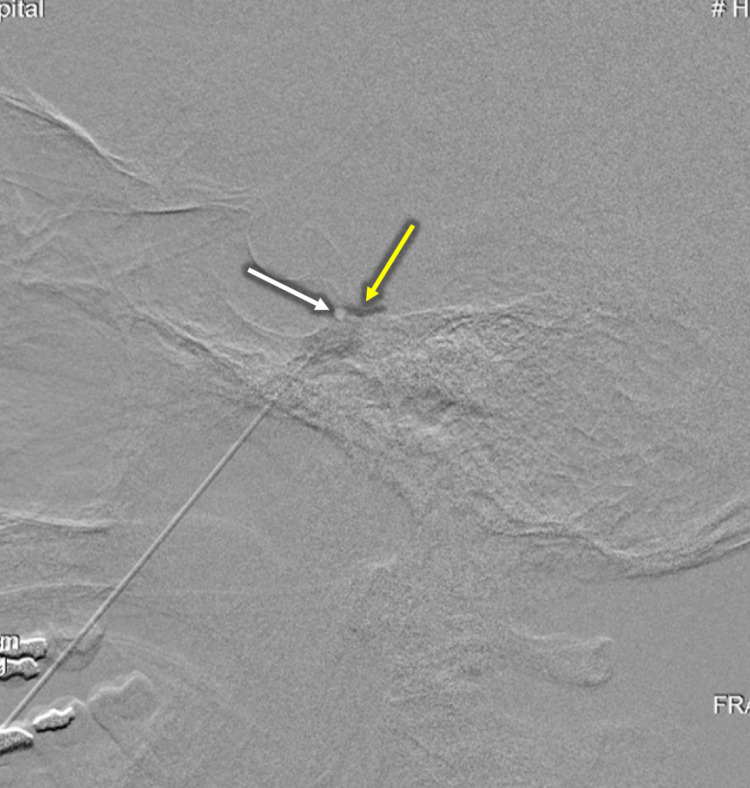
Digital subtraction CT for needle confirmation Contrast injection used to identify needle tip placement (white arrow) within opacified Meckel’s cave (yellow arrow).
